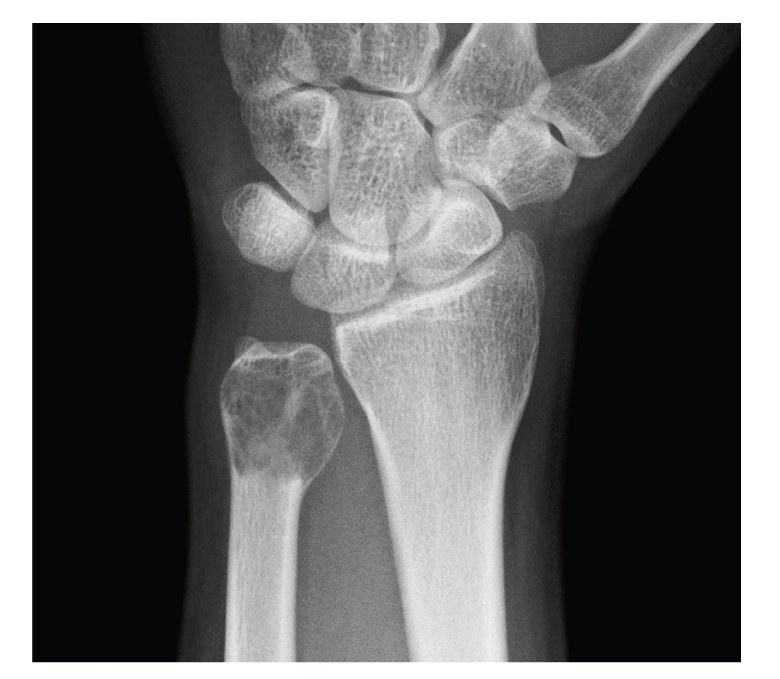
Preoperative plain X-ray showed an expansile, multilobular, and radiolucent lesion with a clear margin in the distal end of the left ulna.
